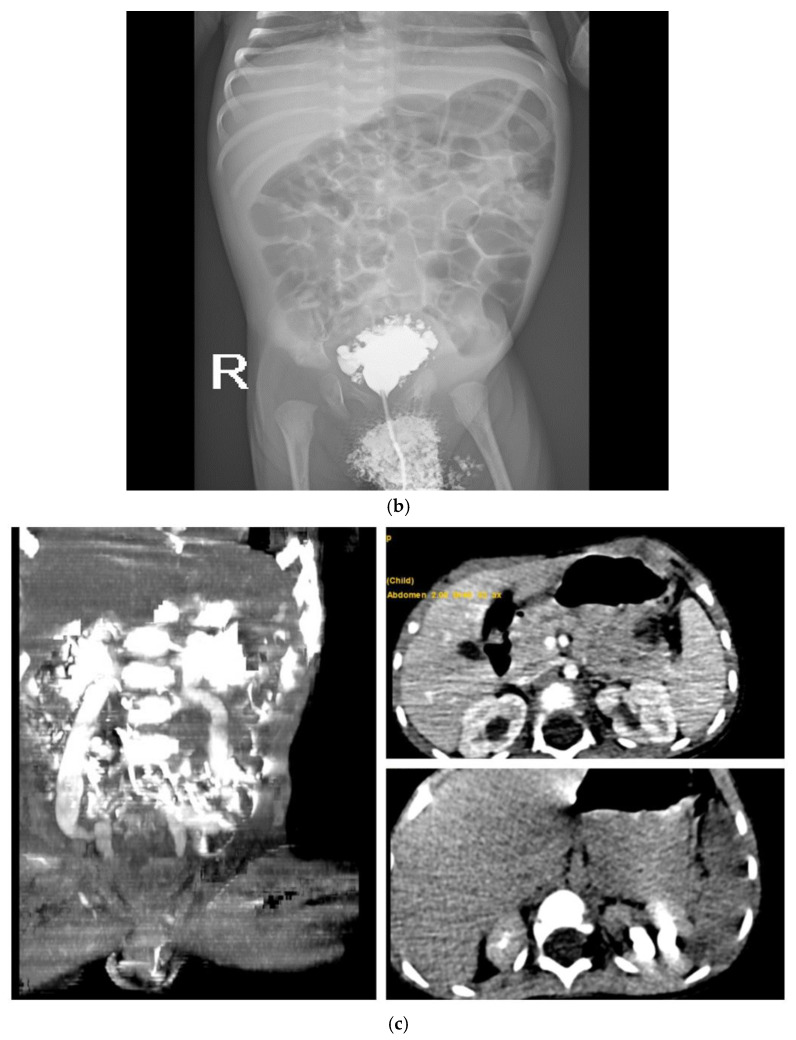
Imaging findings: (a) Bilateral Hydronephrosis with Hydroureters on Ultrasound; (b) voiding cystourethrography—bladder diverticulosis, no signs of vesicoureteral reflux; (c) CT scan of UTS—Bilateral Hydronephrosis with Hydrourethers.
